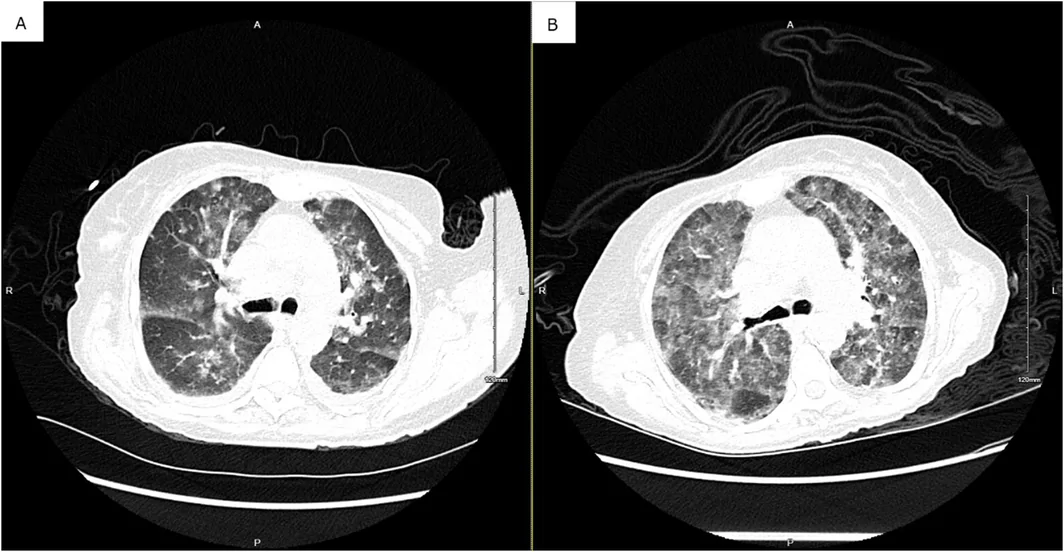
Chest computed tomography findings. (A) Chest computed tomography obtained during the initial hospitalization demonstrated patchy bilateral ground‐glass opacities. (B) Repeat chest computed tomography during the second hospitalization showed interval progression with more extensive bilateral ground‐glass opacities.
